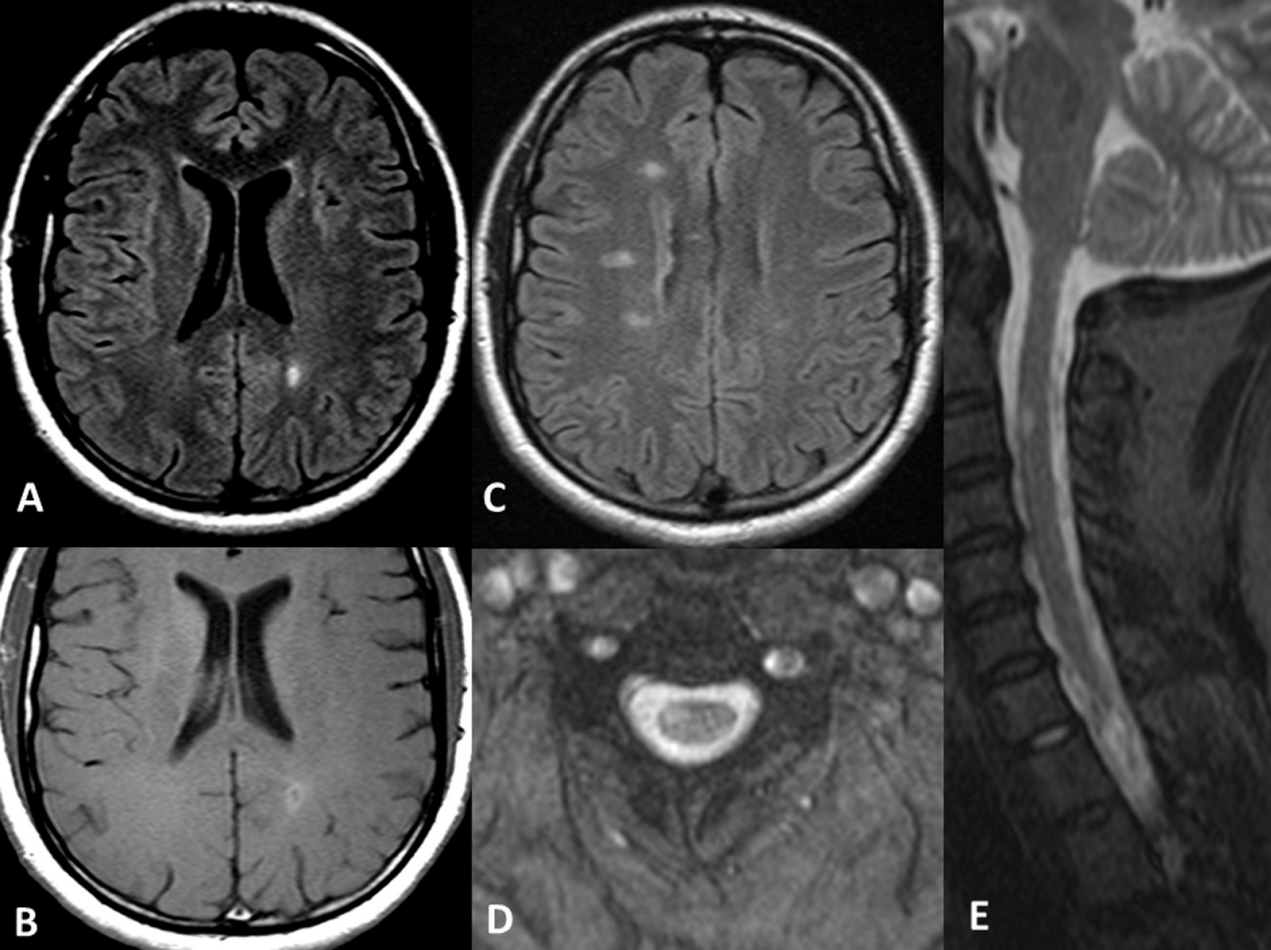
A focal subcortical hyperintense FLAIR lesion (
